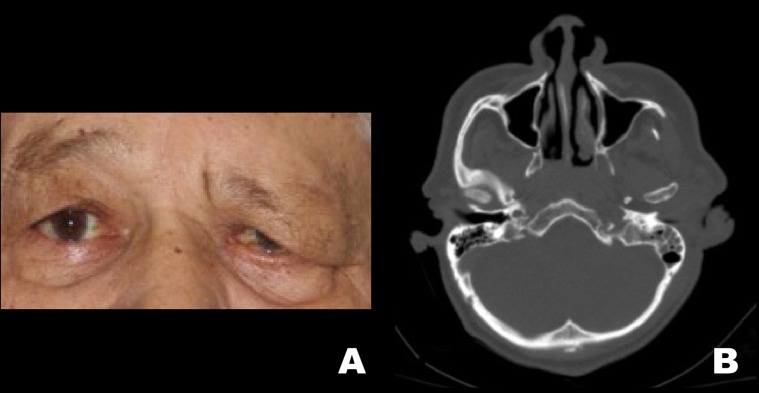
A. Extraoral clinical image of the left eyelid ptosis. B. Axial CT (bone-window display) showing a thickening of the left pinna and external ear conduit.
